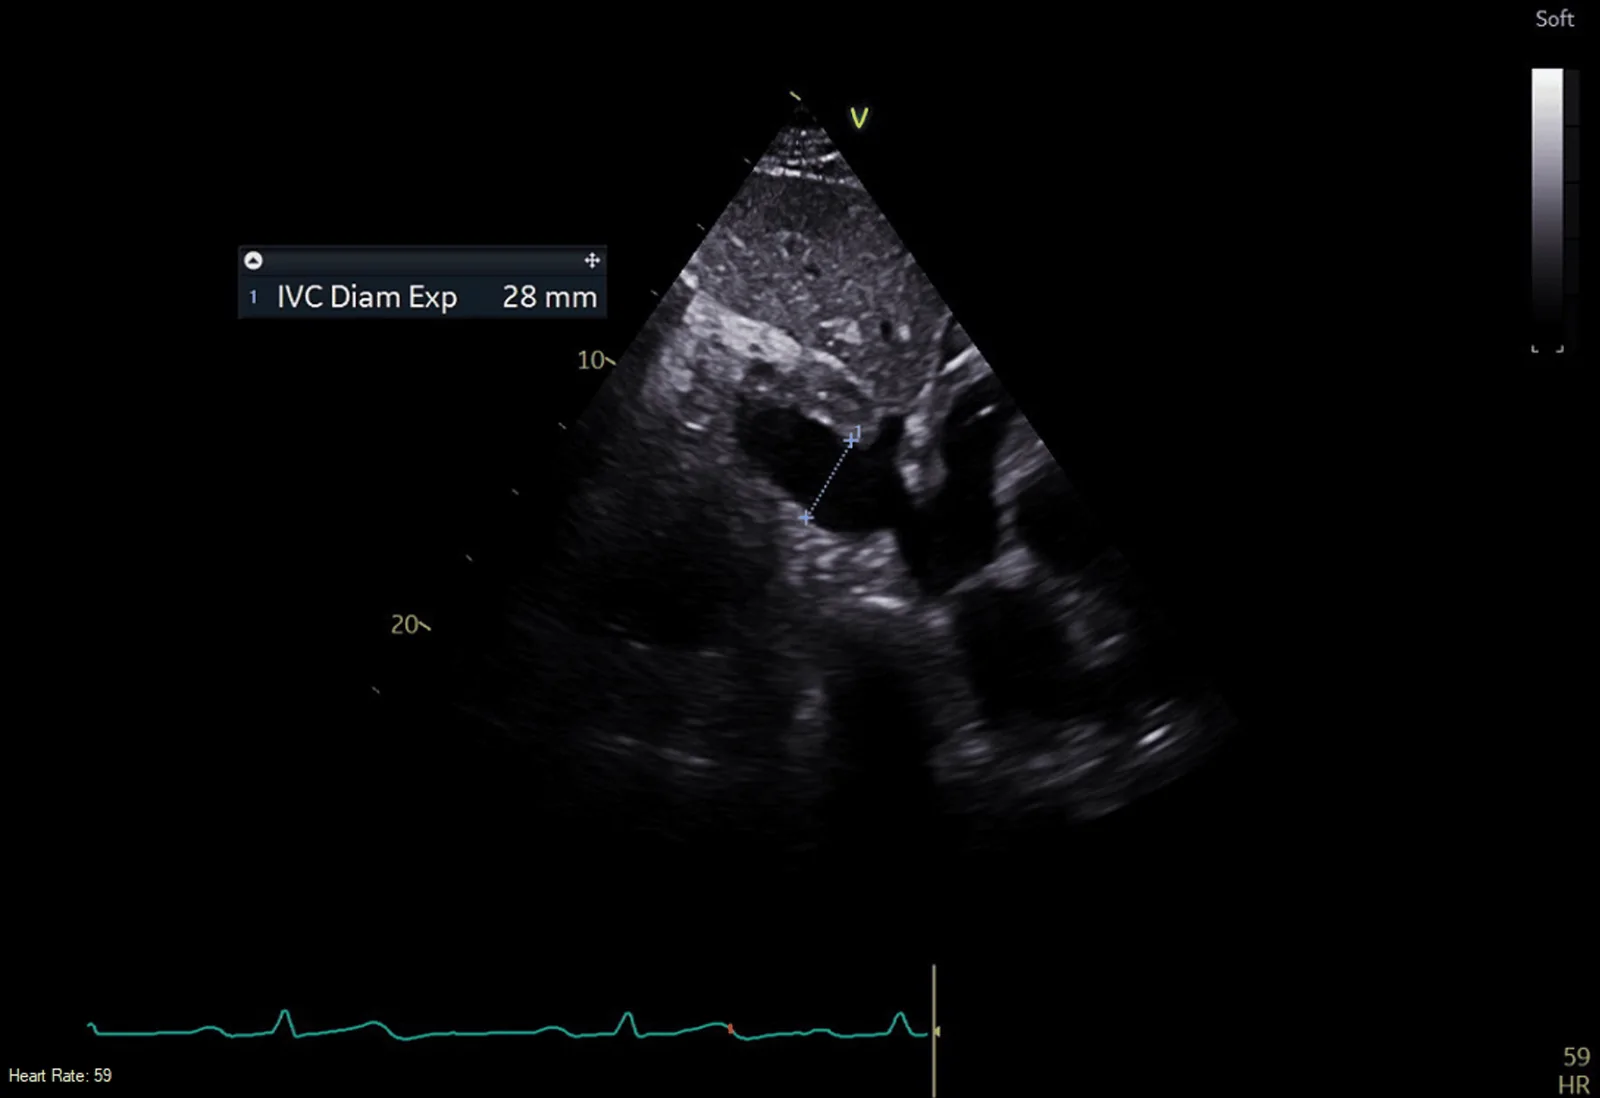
Transthoracic echocardiography (TTE) image showing plethoric inferior vena cava (IVC).
